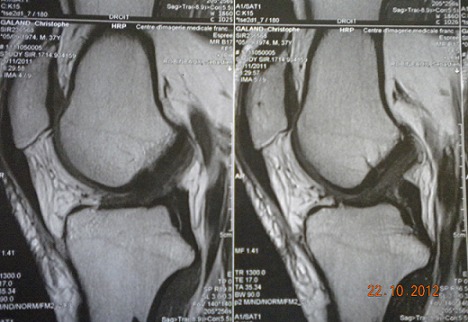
Sagittal T1-weighted image showing inhomogenous high signal intensity of the intact ACL.
